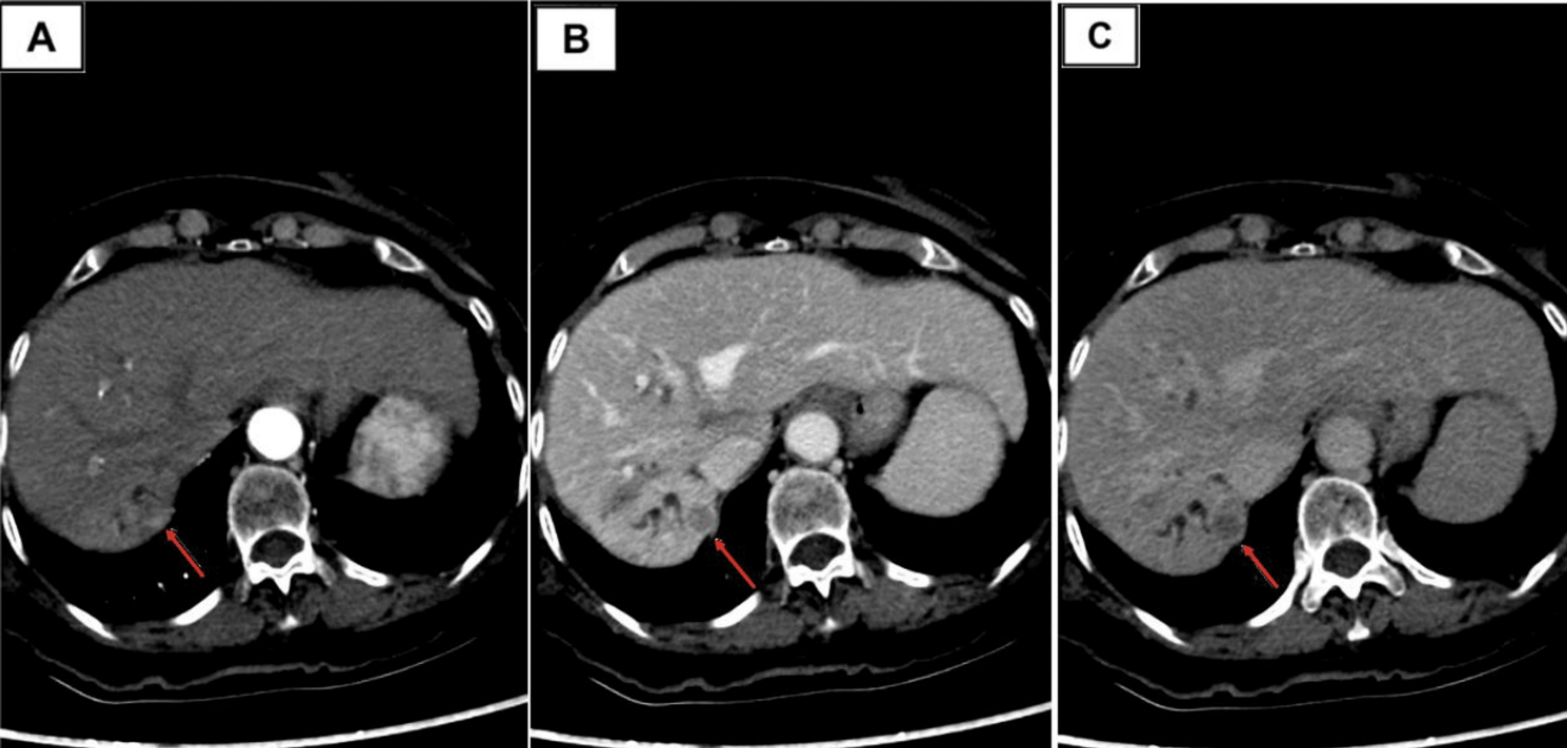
Triphasic liver protocol CT with IV contrast demonstrating an arterially enhancing lesion in hepatic segment VII (red arrows). (A) Arterial phase image shows a subtle hyperenhancing focus measuring 11 mm. (B) In the portal venous phase, the lesion becomes hypoattenuating relative to the background liver, consistent with washout. (C) Delayed phase demonstrates persistent washout with a thin peripheral pseudocapsule. The overall enhancement pattern is diagnostic for hepatocellular carcinoma (LI-RADS 5). LI-RADS: The Liver Imaging Reporting and Data System.
